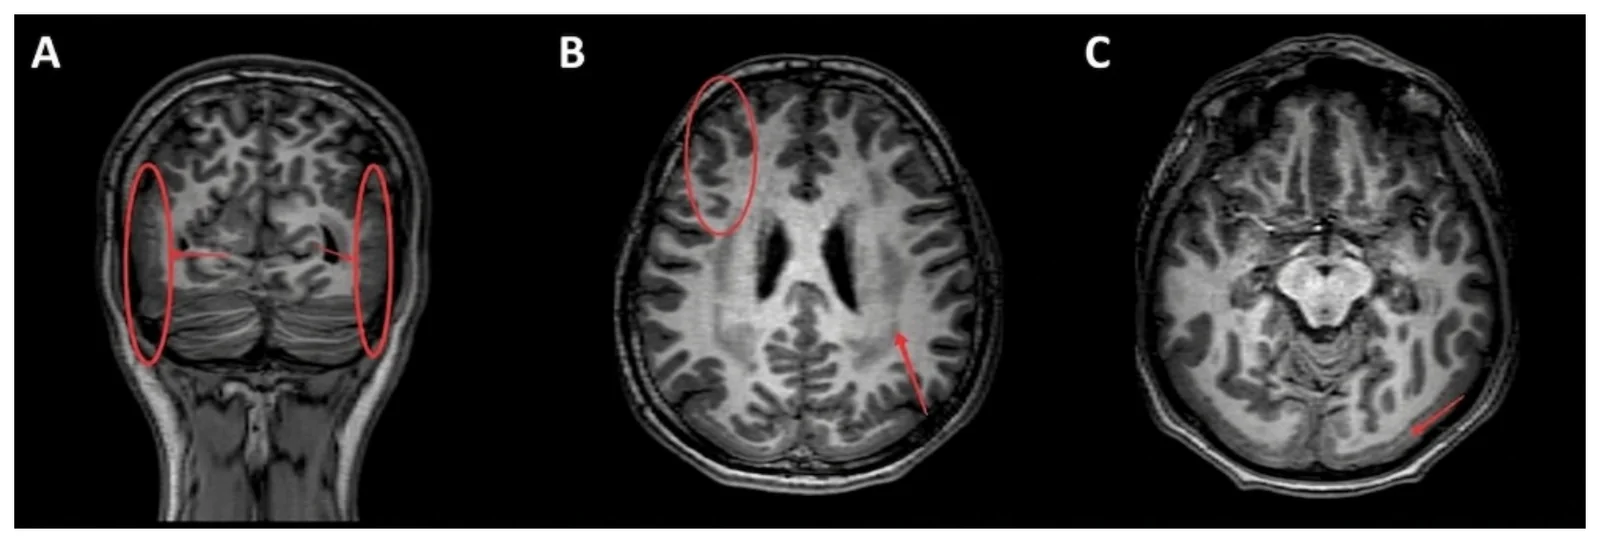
Cranial Magnetic Resonance Imaging (MRI) demonstrating complex malformations of cortical development, subcortical leukodystrophy-like changes, and subcortical U-fiber preservation. (A) Coronal T1-weighted image demonstrates bilateral symmetrical subcortical band heterotopia (red ovals) beneath the thickened cortex, accompanied by confluent subcortical white matter signal alterations mimicking a leukodystrophic pattern. (B) Axial T1-weighted image at the level of the lateral ventricles demonstrates an area of parietal polymicrogyria with irregular, closely packed gyri and shallow sulci overlying subcortical U-fibers that remain well-preserved (red circle), despite extensive adjacent leukodystrophy-like white matter abnormalities and posterior band heterotopia (red arrow). (C) Axial T1-weighted image at the posterior occipitotemporal level highlights marked posterior occipital pachygyria and a contiguous thick layer of heterotopic gray matter beneath the cortex (red arrow), confirming bilateral posterior-predominant band heterotopia (double cortex syndrome).
